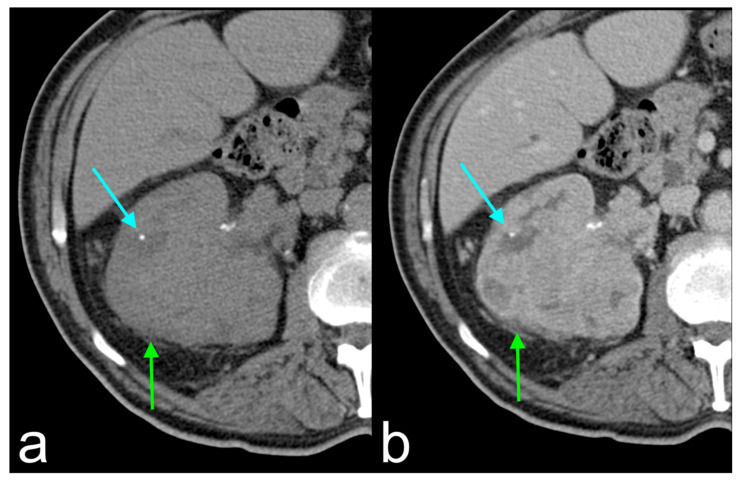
Unenhanced axial CT image (a) and axial CT image during venous phase (b) showing ccRCC with BAP1 gene mutation with ill-defined margins (green arrows) and tumor calcification (light blue arrows). Images from The Cancer Genome Atlas Kidney Renal Clear Cell Carcinoma Collection (TCGA-KIRC); https://www.cancerimagingarchive.net/collection/tcga-kirc/ (accessed on 1 May 2024).
